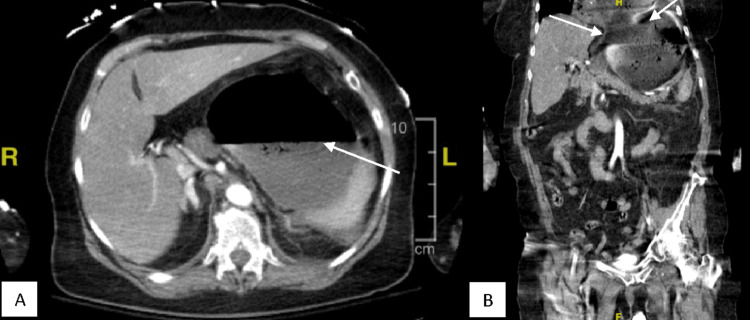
Preoperative CT scans: (A) gastric distension, air-fluid level, and adjacent inflammatory changes concerning for gastric volvulus; (B) paraesophageal hernia.
